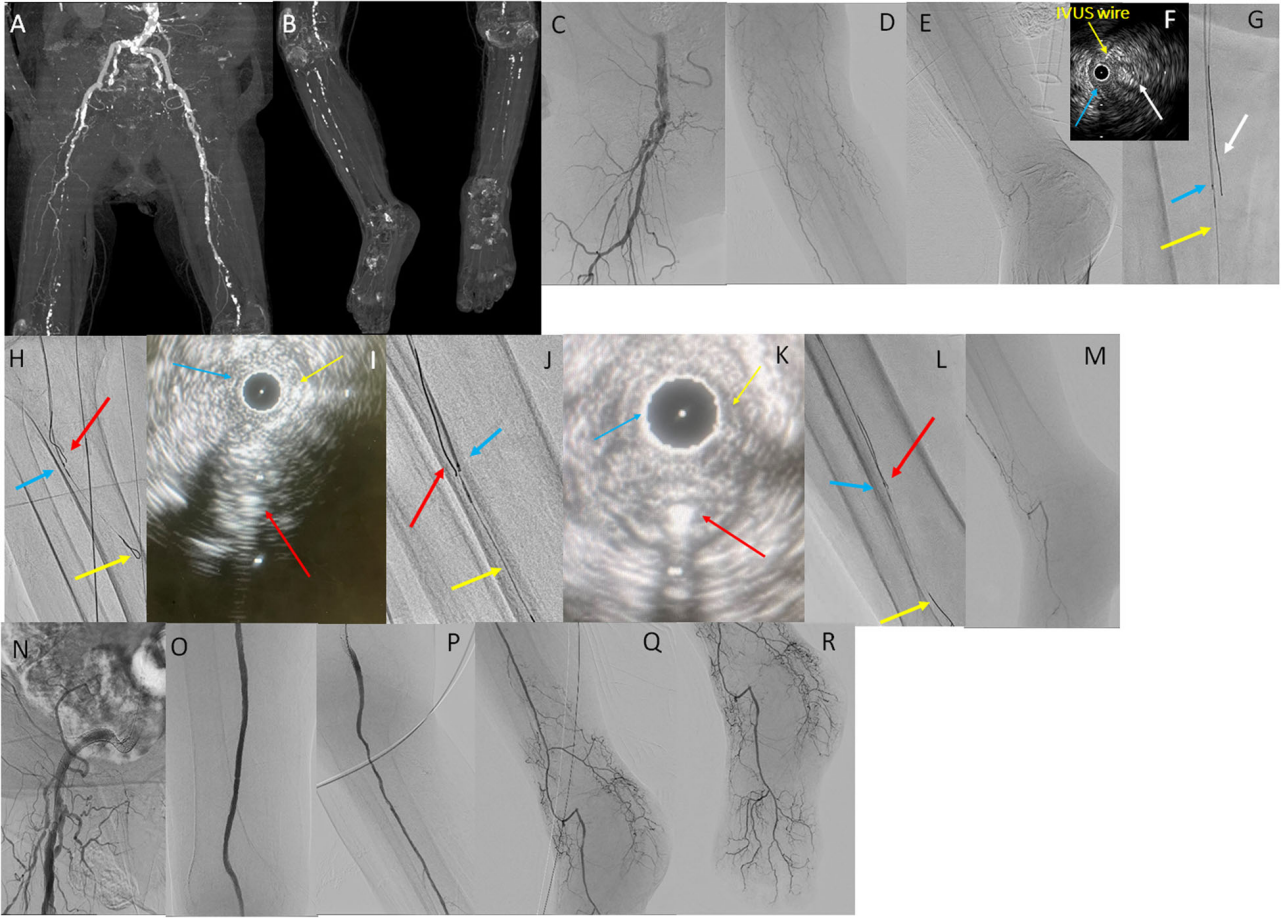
A, B Preprocedural enhanced computed tomography. C, D, E Control angiography shows total occlusion from the right superficial femoral artery (SFA) to below-the-knee arteries. Distal target vessels are very faint. F IVUS findings of the CTO lesion in the SFA. Yellow arrow: IVUS wire; blue arrow: IVUS transducer; white arrow: 2nd GW in the intraplaque route. G Intravascular ultrasound (IVUS)-guided parallel wiring (G: SFA). Yellow arrow: 1st guidewire (GW) and IVUS catheter; blue arrow: IVUS transducer; white arrow: 2nd GW advanced into the intraplaque of the chronic total occlusion (CTO) lesion. H, J, L IVUS-guided parallel wiring (H: distal popliteal artery to peroneal artery; J: peroneal artery; L: distal peroneal artery). Yellow arrow: 1st GW; blue arrow: IVUS transducer; red arrow: 2nd GW advanced into the intraplaque of the CTO lesion. I, K IVUS findings of the CTO lesion in the below-the-knee artery (I: distal popliteal artery to peroneal artery; K: peroneal artery). Yellow arrow: IVUS wire; blue arrow: IVUS transducer; red arrow: 2nd GW in the intraplaque. M Tip injection from the distal peroneal artery shows the distal true lumen of below-the-ankle arteries. N, O, P, Q, R Final angiography shows sufficient antegrade blood flow
